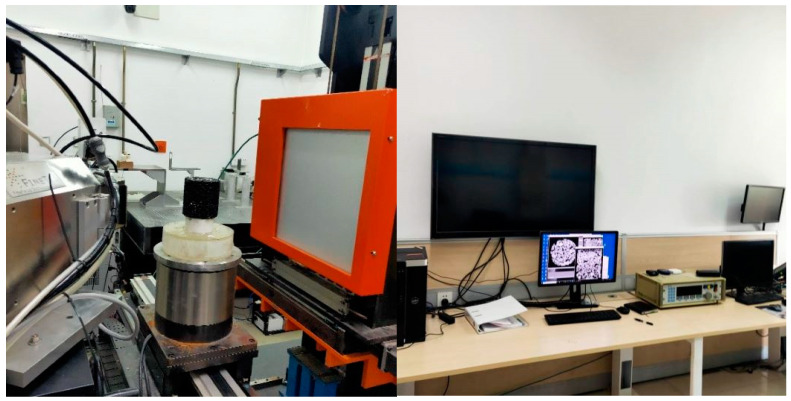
Industrial CT scan test instrument charts.
